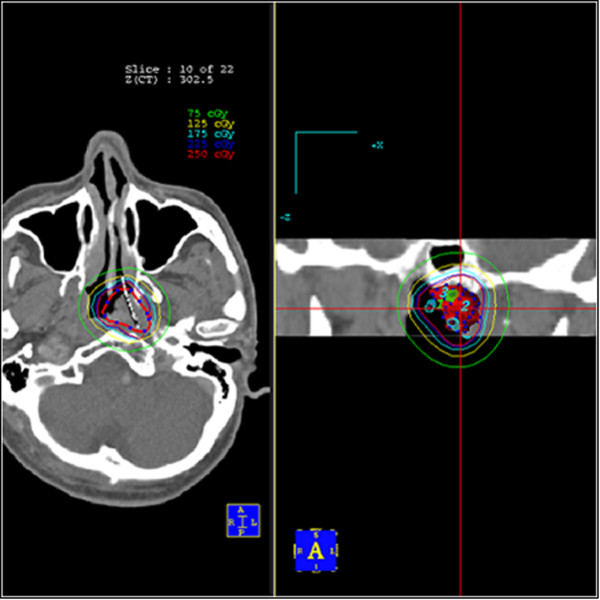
Axial computed tomography (CT) image of a stage T1-2a NPC patient showing two brachytherapy intracavitary applicators
